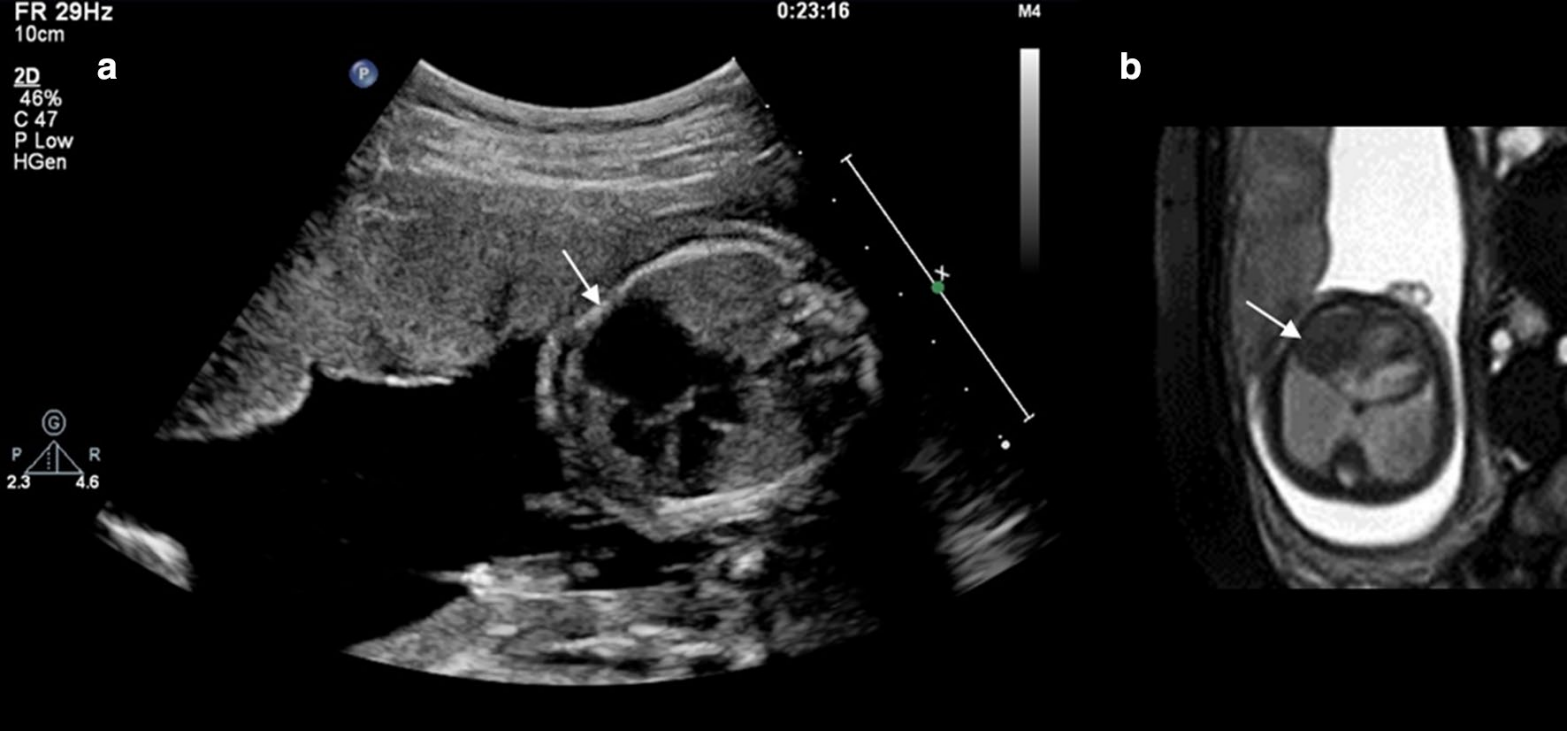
A 25-week fetus with Ebstein anomaly. Fetal echocardiography and CMR B-TFE four-chamber view image shows the flow regurgitation is from right ventricle to right atrium and significantly dilated right atrium (*arrows* in a and b)
